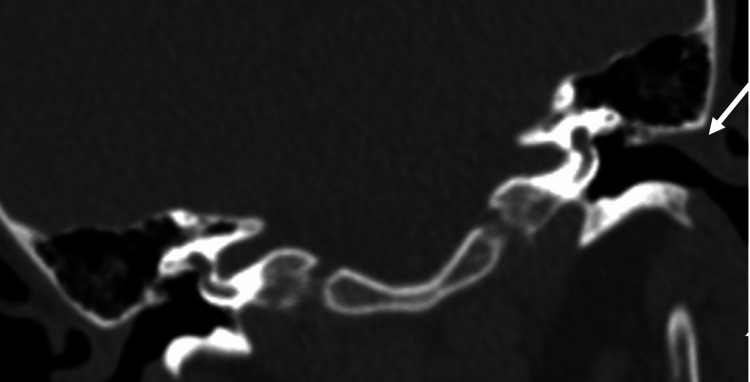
Coronal cut of high-resolution computed tomography (CT) scans through the temporal bones when the patient was age four. Soft tissue obstruction of the left external auditory canal (EAC) is noted (arrow).
